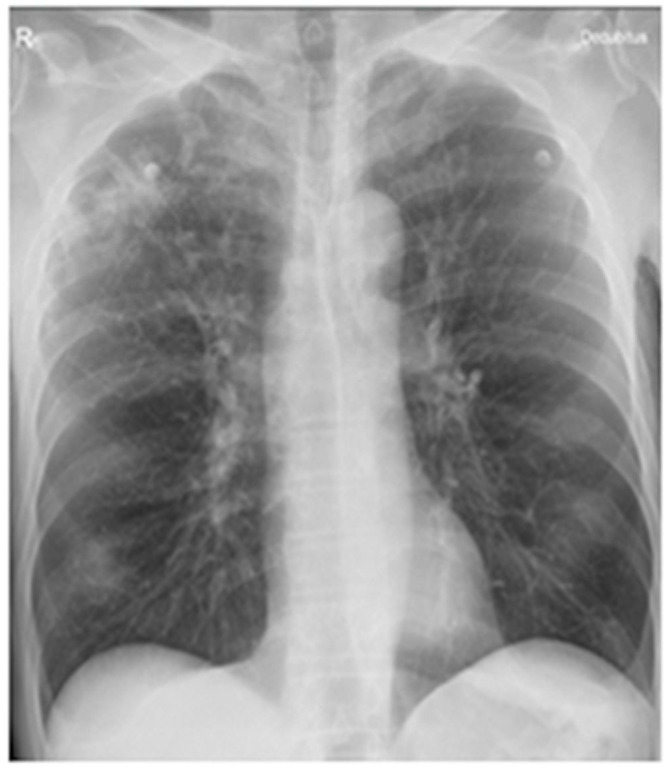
Chest X-ray from 17 December 2023, shows new external subclavicular nodular infiltrative lesions in the right lung and multiple diffusely defined ground-glass opacities in both lung bases.
